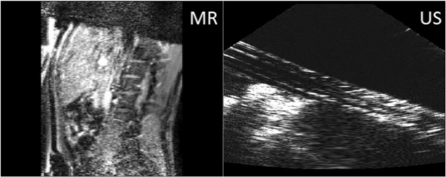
Sagittal images of the liver and the upper intestinal tract of a volunteer with T2*-weighted MRI on the left and tissue harmonic imaging ultrasound on the right
